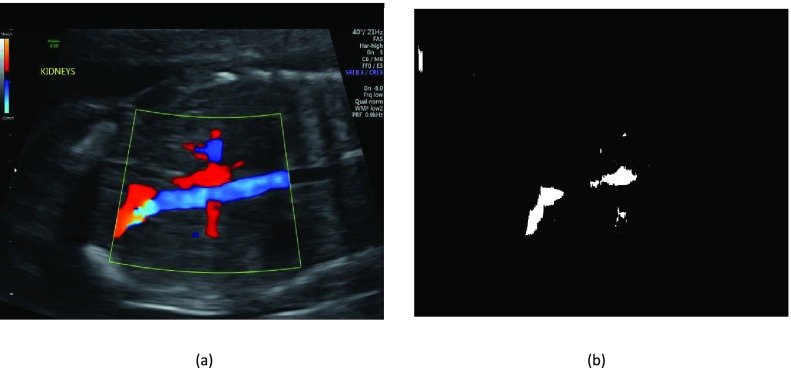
(a) A color Doppler image, and (b) its thresholded mask.
